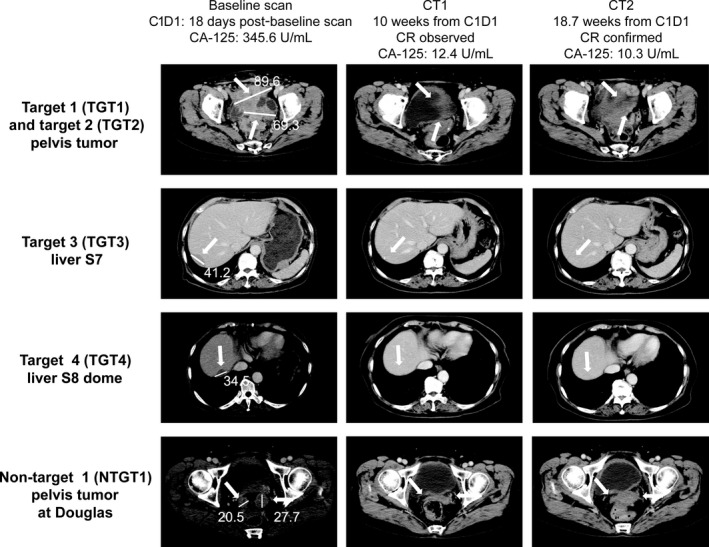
Computed tomography images showing a complete response to veliparib 100 mg b.i.d. plus carboplatin and paclitaxel in a single patient. C1D1, cycle 1, day 1; CA‐125, cancer antigen 125; CR, complete response; CT, computed tomography.
